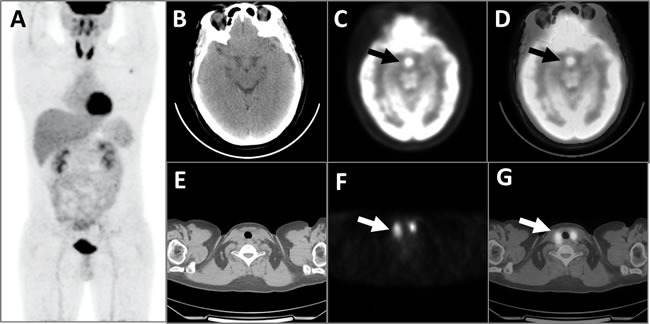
This patient with LCH had concurrent diffuse thyroid hypermetabolism The MIP image of the PET A. showed diffuse abnormal FDG hypermetabolism with an SUVmax of 11.3 in the residual bilateral thyroid, which was consistent with the CT scanning E., PET cross-section images F., and fusion images G. of the thyroid (white arrows). PET images of the brain B. showed significantly elevated FDG metabolic activity with an SUVmax of 12.9 (black arrows) in the pituitary, which was confirmed by the corresponding CT C. and fusion D. images.
